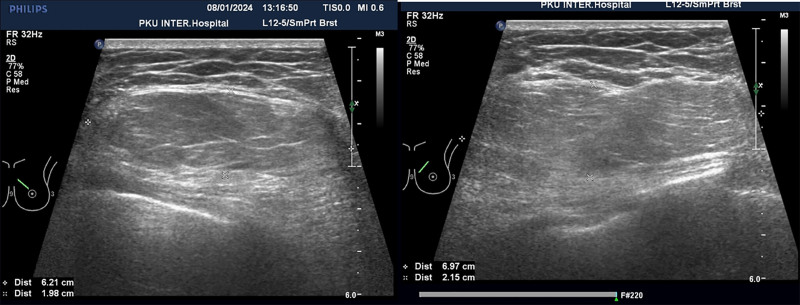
Ultrasound examination showed a large mass in the left breast.
